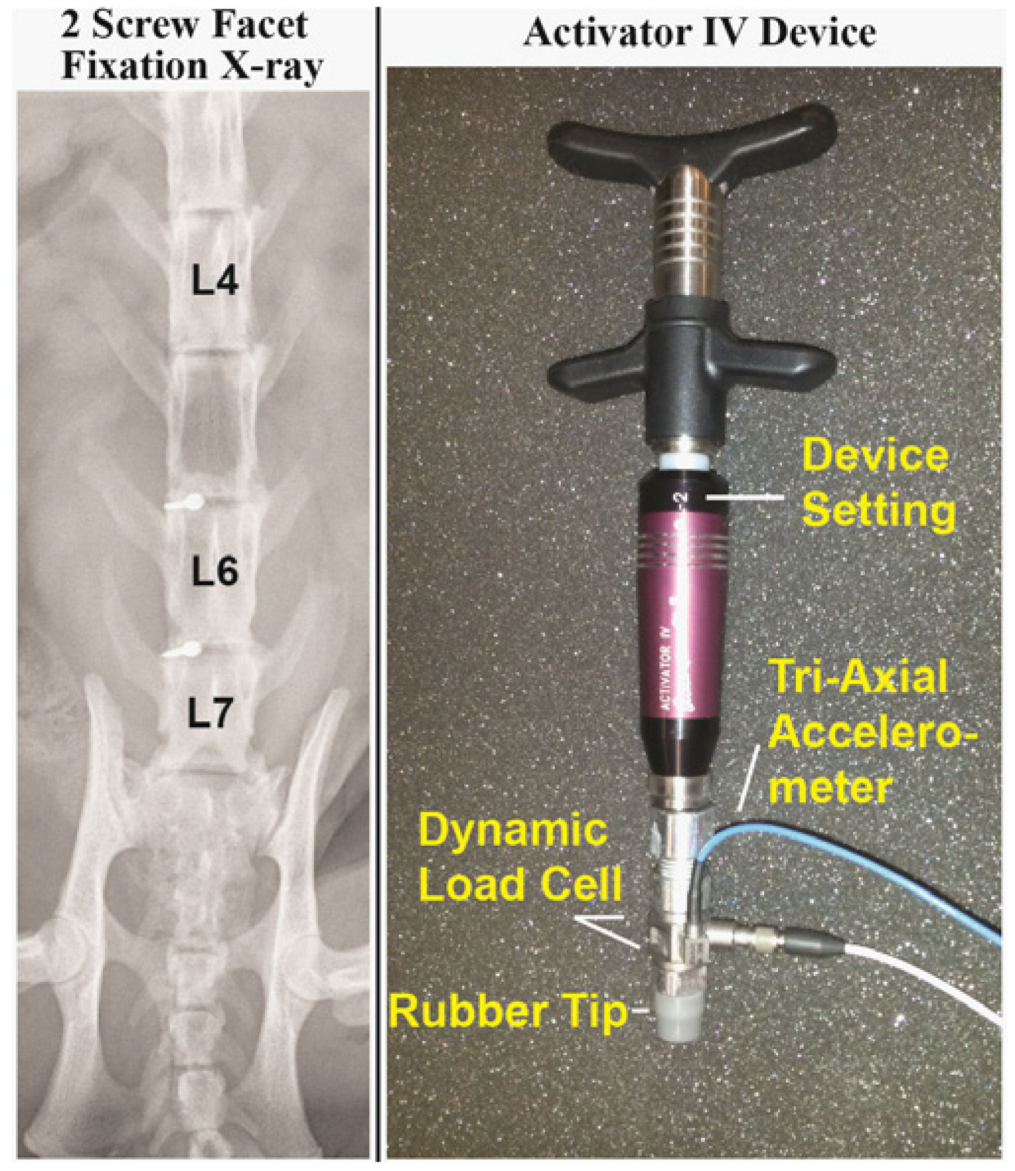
An x-ray of the unilateral L5-6 and L6-7 facet joint fixation and a photograph depicting the modified Activator IV device with attached dynamic load cell and tri-axial accelerometer.
